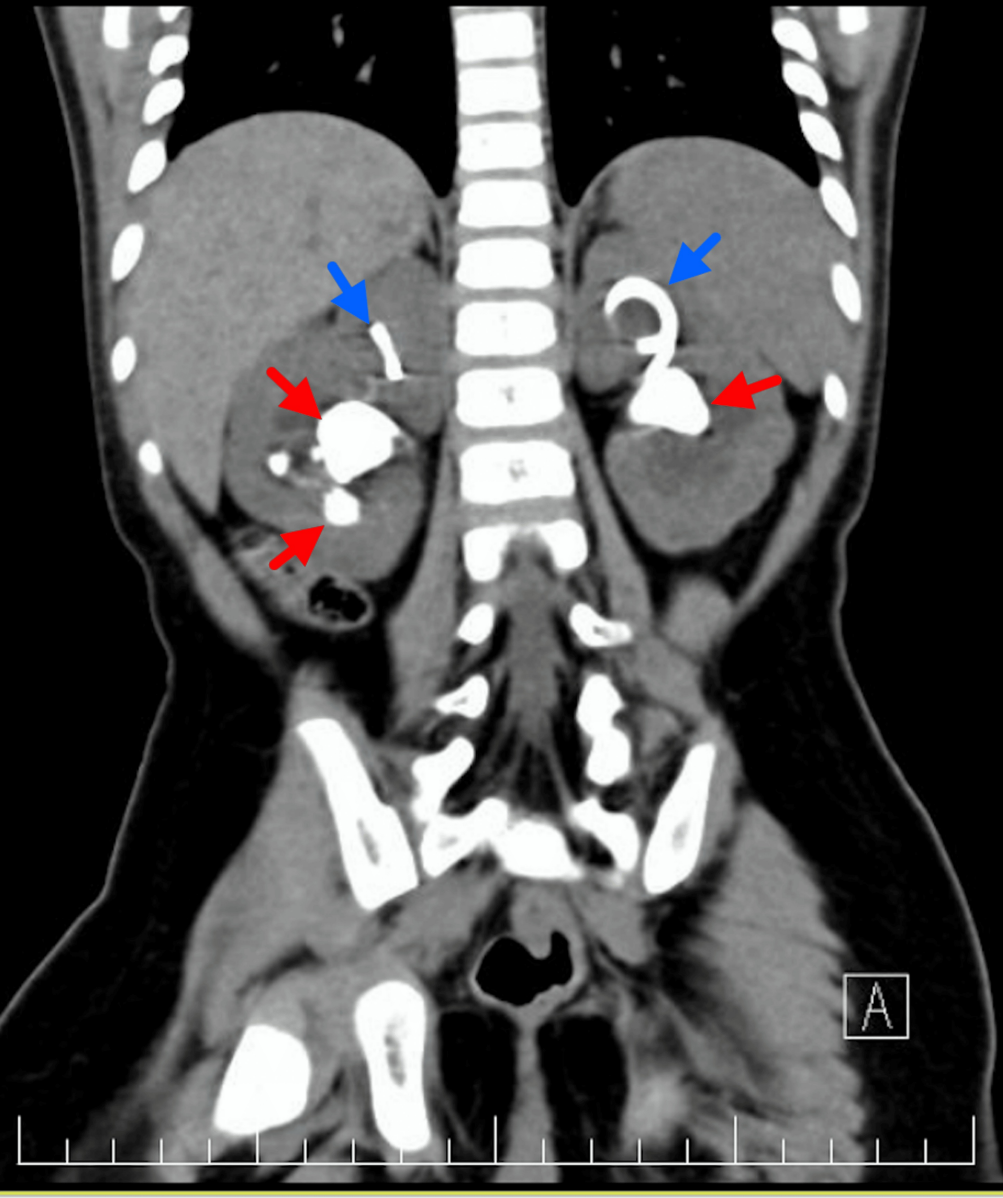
Coronal view of non-contrast-enhanced CT of the abdomen and pelvis Bilateral staghorn calculi are indicated by the red arrows, and the renal tips of the double-J (DJ) stents are indicated by blue arrows.
